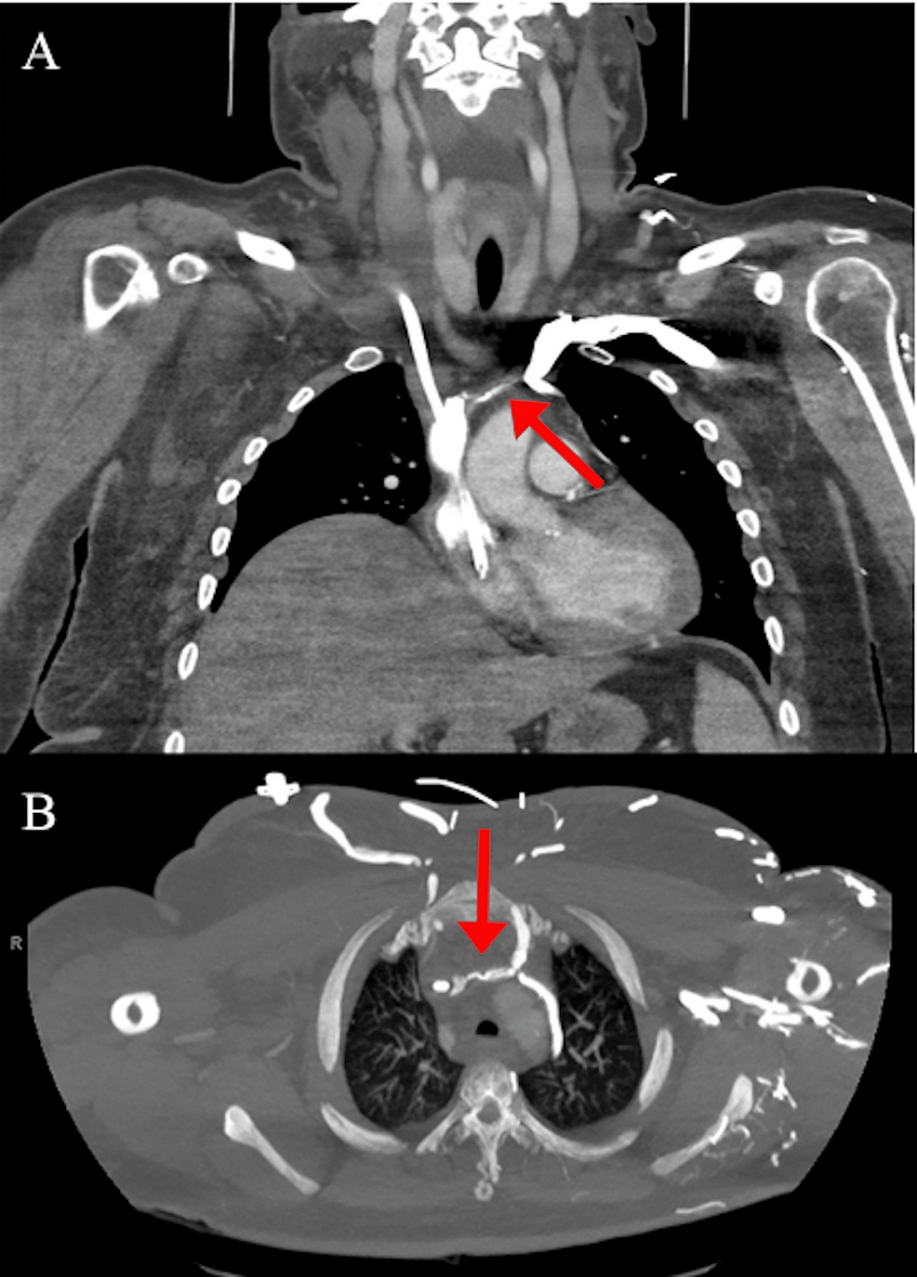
Computed tomography (CT) scan of chest and neck with brachiocephalic vein occlusion (red arrows)
